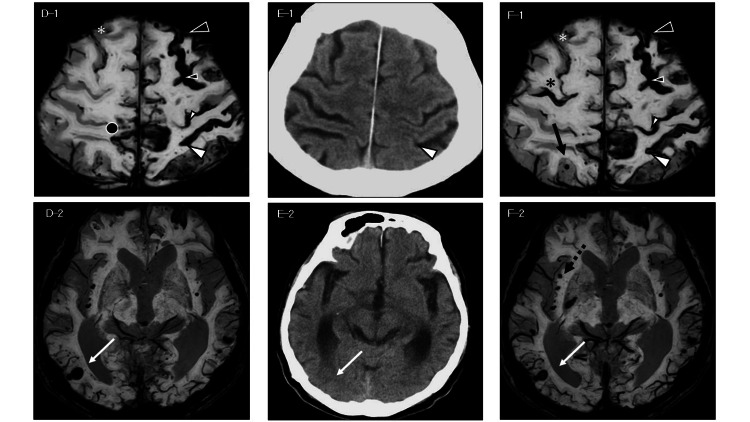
Follow-up imaging from seven to 10 months in the patient with probable CAA with APOE ε2/ε2 D-1: The SWI two days later reveals a single-line hypointense signal in the left postcentral sulcus (white arrowhead) suggesting acute cSAH. In addition, a new cSAH is observed in the right parietal lobe, adjacent to a pre-existing cSAH (black circle); D-2: A new ICH is noted in the right occipital lobe (white arrow) on SWI; E-1, E-2: Follow-up CT showed resolution of the previously identified hyperdense lesions; F-1: The SWI at 10 months demonstrates a distinct double-line hypointense signal in the left postcentral sulcus (white arrowhead), consistent with cSS. A new cSAH is seen in the right frontal lobe (black asterisk), along with a new CMB in the right parietal lobe (black arrow); F-2: An additional CMB appears near the external capsule in the right hemisphere (black dotted arrow) Black arrowhead: Anterior portion of the left superior frontal sulcus; Small black arrowhead: Posterior portion of the left superior frontal sulcus; White arrowhead: Left postcentral sulcus; Small white arrowhead: Left central sulcus; White asterisk: Anterior portion of the right superior frontal sulcus; White arrow: Hemorrhagic foci in the right occipital lobe. Symbols are consistent across D–1 to F–2. SWI: Susceptibility-weighted imaging; ICH: Intracerebral hemorrhage; cSAH: Convexity subarachnoid hemorrhage; cSS: Cortical superficial siderosis; CMB: Cerebral microbleed; CAA: Cerebral amyloid angiopathy; APOE: Apolipoprotein E
